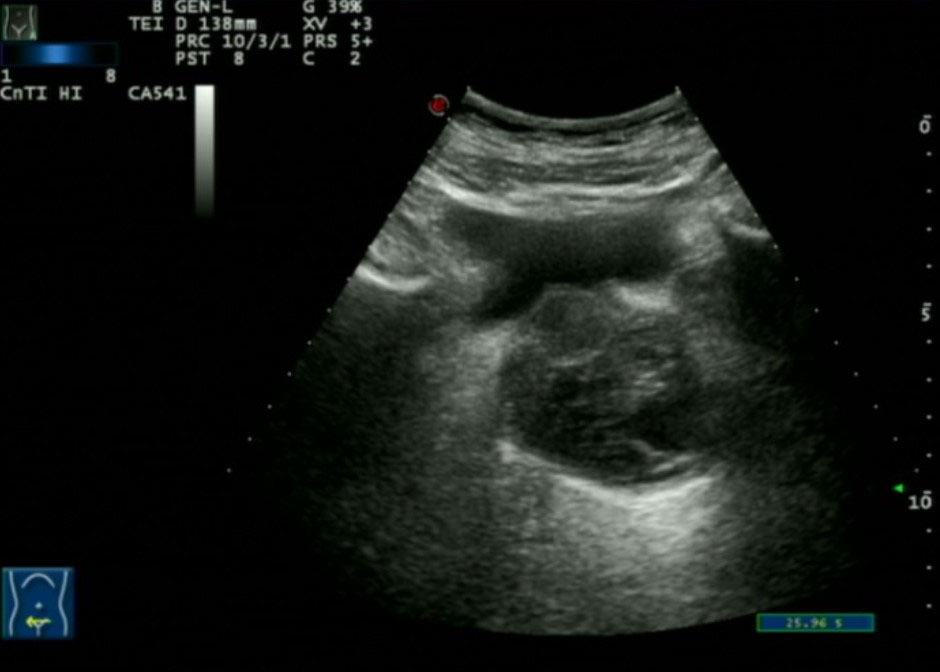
Prostate ultrasound showing an enlarged prostate with an intact capsule. The parenchyma exhibits heterogeneous echogenicity with patchy areas of strong echoes. A cystic-solid mixed echogenic mass, measuring approximately 5.0 x 4.8 cm, with unclear boundaries and heterogeneous internal echoes, is observed within the prostate.
